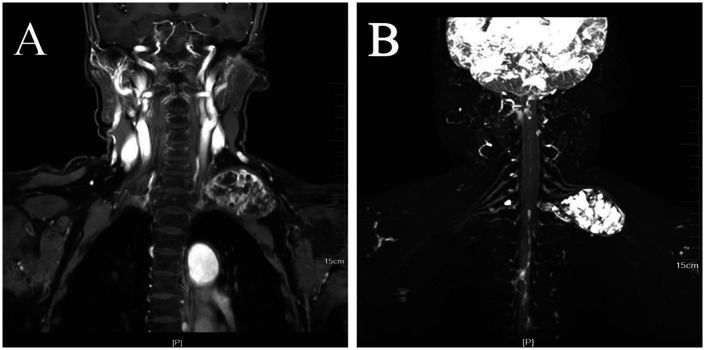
Images from different imaging sequences. (A) Conventional magnetic resonance imaging (MRI) sequence images. (B) 3D-STIR-SPACE sequence better exposed the tumor morphology.
